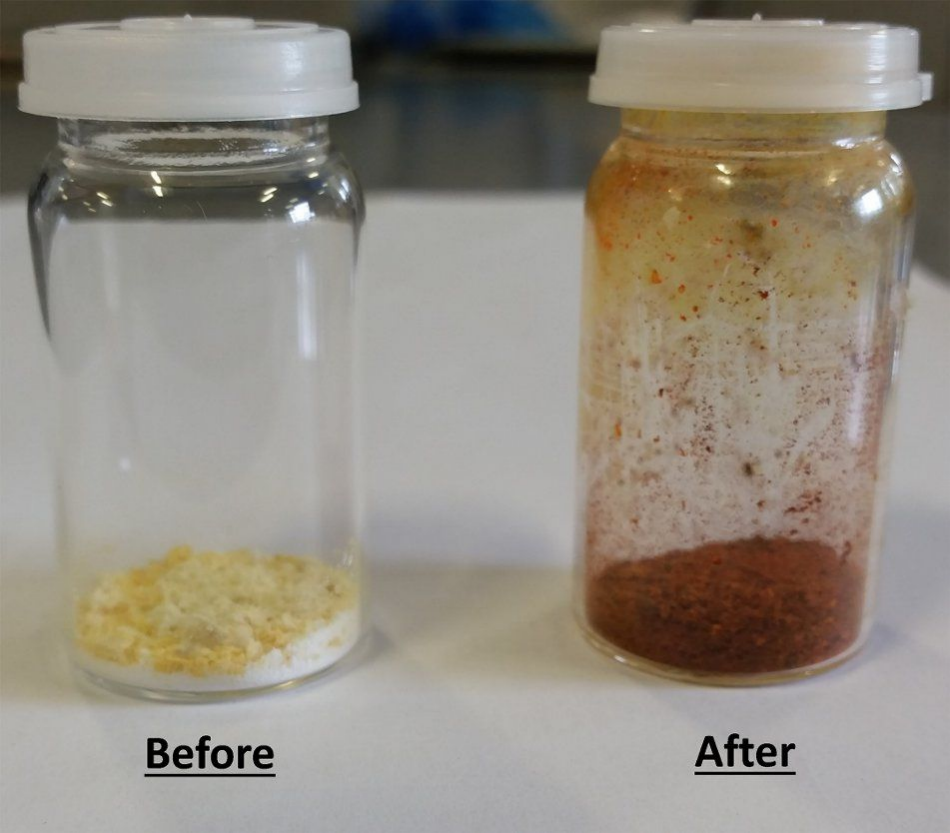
Reaction mixture before and after ultrasonic irradiation for 60 minutes.
